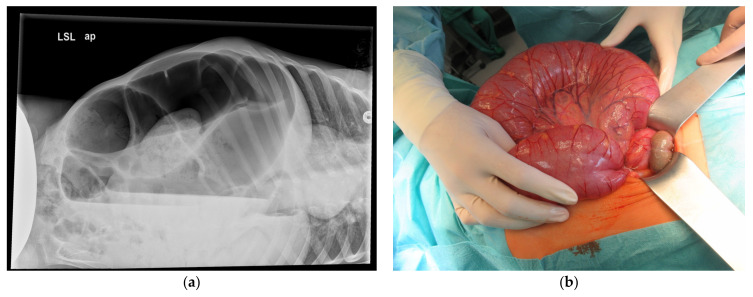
Patient 1, acute sigmoid volvulus: (a) lateral decubitus radiograph with ‘coffee-bean-sign’; (b) corresponding intraoperative finding.
